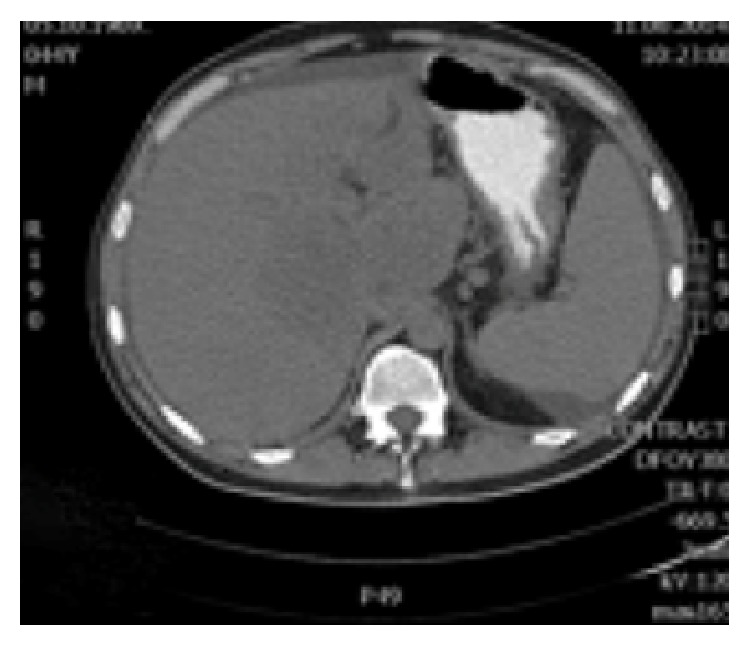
On noncontrast CT phase lesion was poorly visualized, shaped as a flower with a lower density than liver parenchyma.
